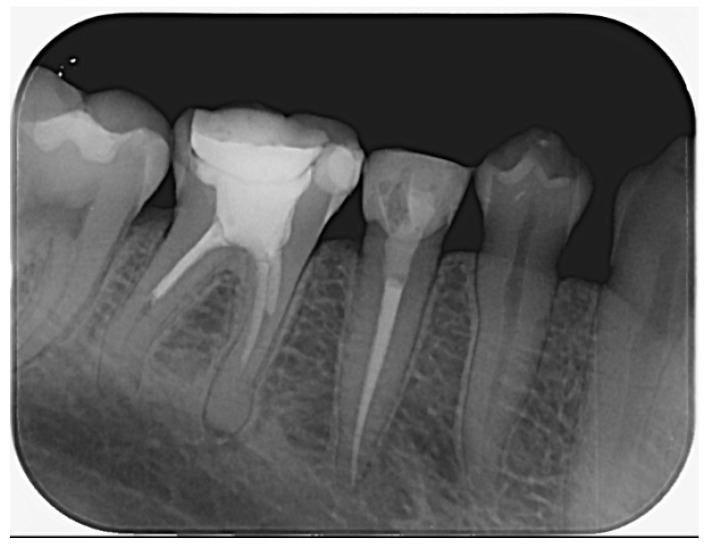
Clinical and radiographic follow-up of tooth 45 at 2.5 years post-endodontic retreatment and postless restoration.
